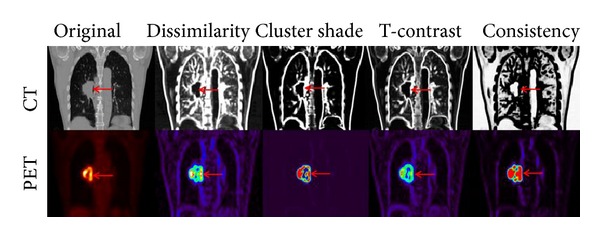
Examples of the different texture maps derived from SGLDM and NGTDM features for coronal CT and PET images of the same patient.
